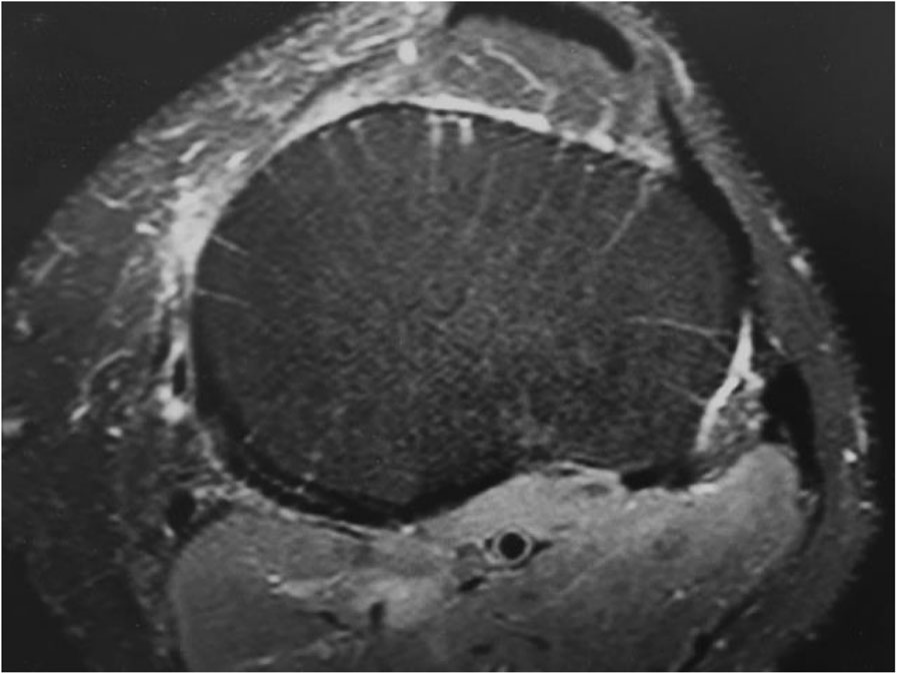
Example of upper tibial axial MRI slice. A typical axial upper tibial PD_SPAIR MRI slice at level 3. White radial vascular marks are evident
